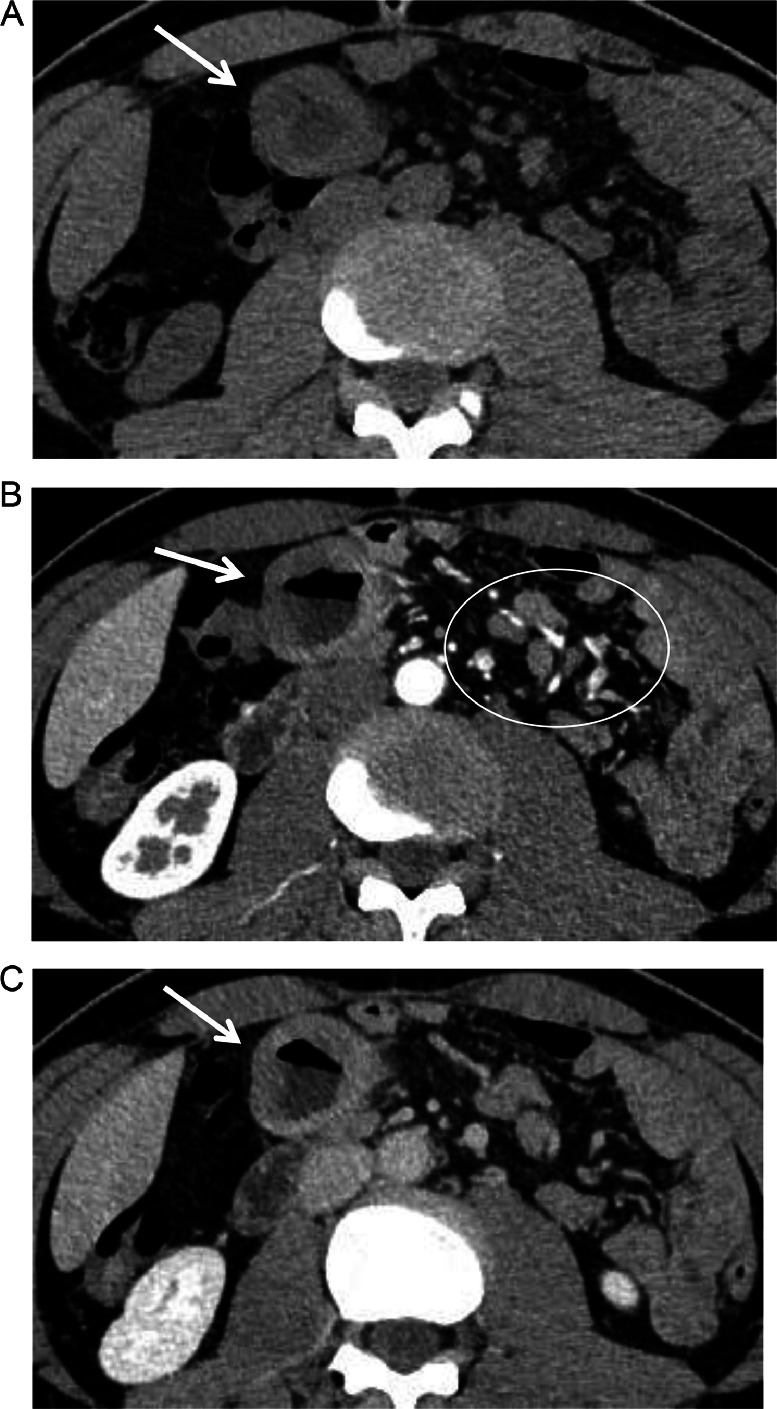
Dynamic CT images of the small intestine. Dynamic CT axial images (A-C) show a small aneurysmal dilatation of the jejunum with homogenous wall enhancement (white arrows). Mesenteric lymph node enlargement is observed (circle in B). A) Pre-contrast phase; B) early arterial phase; C) delayed phase.
